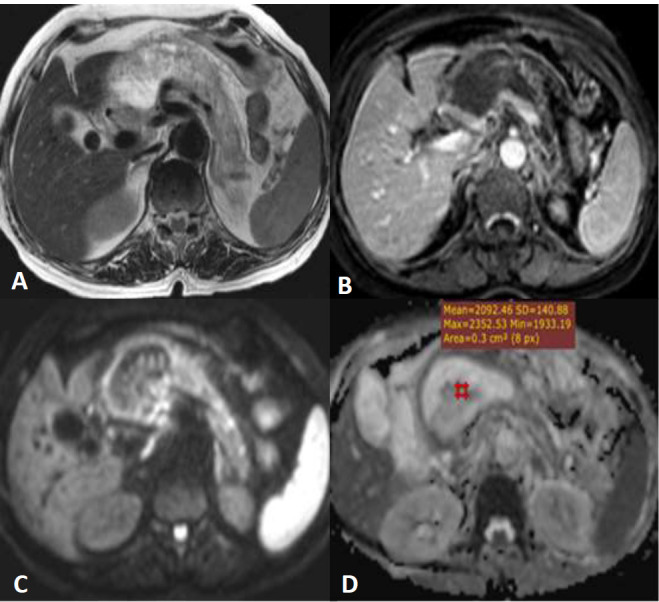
A 46-year-old female patient presented with intense epigastric pain and vomiting, accompanied by elevated serum amylase levels. (A) T2WI showed a hyperintense signal of the pancreatic body. (B) Enhanced T1WI fat sat shows no enhancement of the pancreatic body. (C & D) show facilitated diffusion of the pancreatic body at DWI B 800 and ADC map with ADC value =2.092 X 10-3mm^2^/sec. The case was diagnosed as necrotizing pancreatitis.
